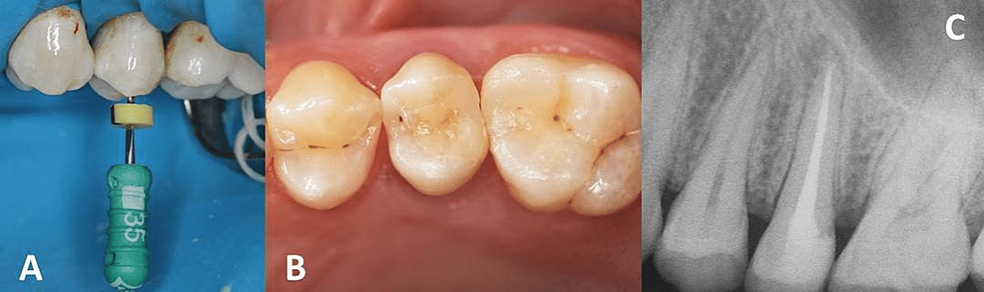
(A) Preparation of the root canals, (B) definitive post-endodontic restoration of the occlusal face, and (C) final radiography to verify the quality of the endodontic and restorative treatment.
